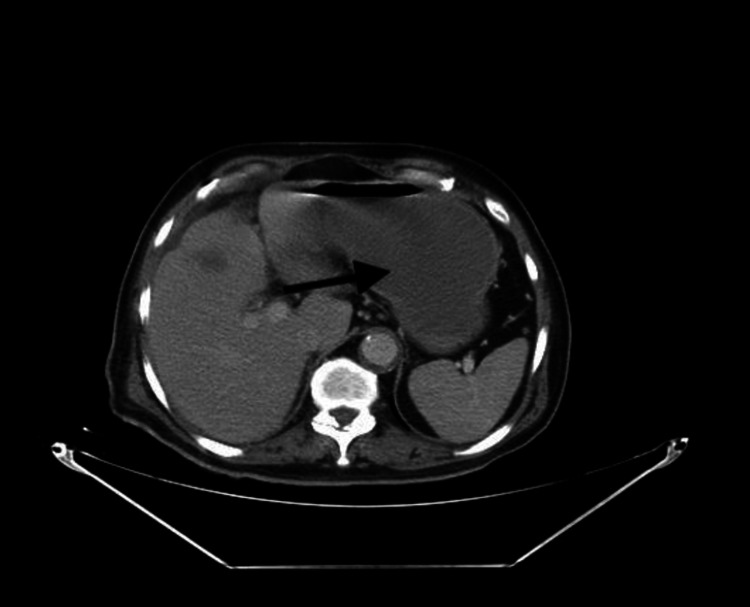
Abdominal CT scan showing distended stomach (arrow) 10 days post-operatively
